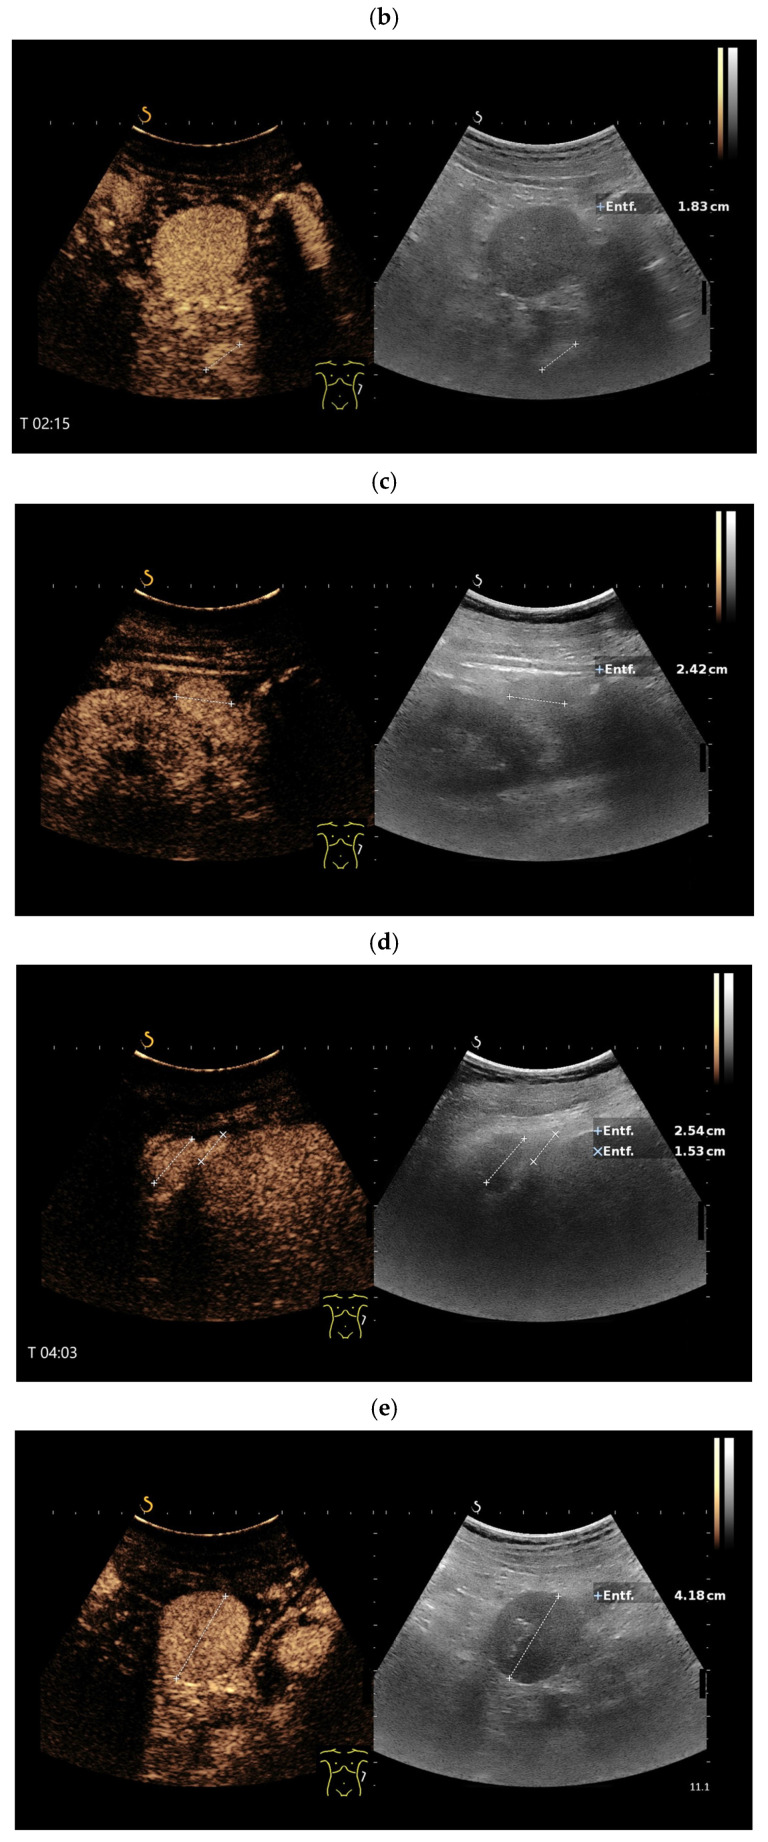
Splenosis. In a patient with a history of splenectomy, a 46 mm smooth-bordered hypoechoic lesion (between the markers) with echogenic internal reflections is visible in the left upper abdomen (a). On CEUS, the lesion shows a homogeneous and prolonged enhancement (b). Additionally, further contrast-enhancing lesions (between the calipers) are also visible, which were previously not discernible in B-mode ultrasound (c–e). Hyperenhancement was visible for longer than 5 min. The figure demonstrates the prolonged enhancement of the lesions; here at 4:23 min (e). Entf. = distance.
